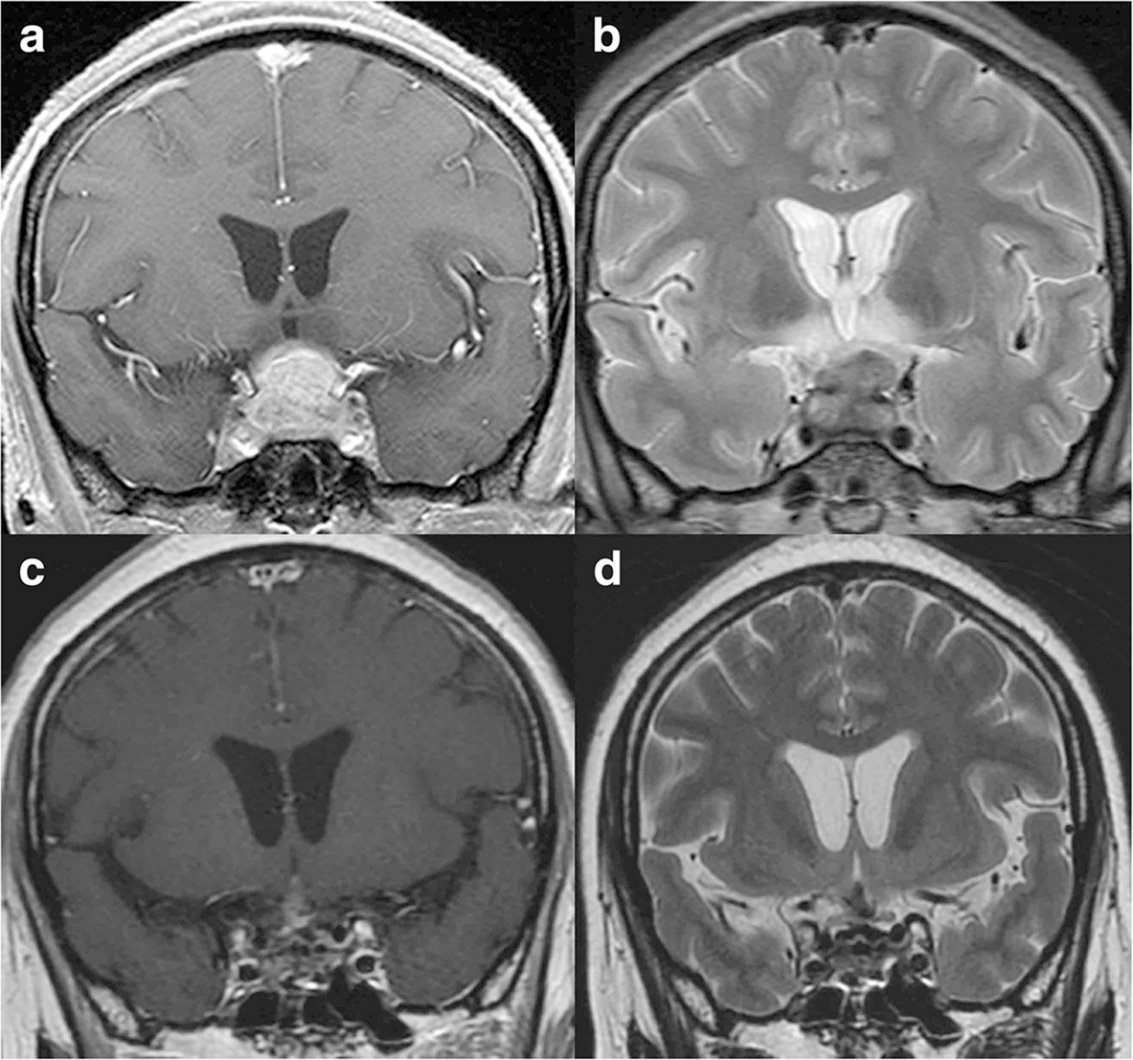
Chronological MR images of Case 1. (a) Preoperative coronal T1-weighted MR image with gadolinium revealing a sellar lesion extending to the suprasellar cistern and bilateral cavernous sinuses. (b) Preoperative coronal T2-weighted MR image revealing a sellar lesion extending to the suprasellar cistern and a vast irregular high intensity area in the bilateral hypothalami. (c, d) Coronal T1-weighted MR image with gadolinium (c) and T2-weighted MR image (d) showing significant reduced volume of the lesion and disappearance of abnormal intensity in the bilateral hypothalami at 13 months after operation.
